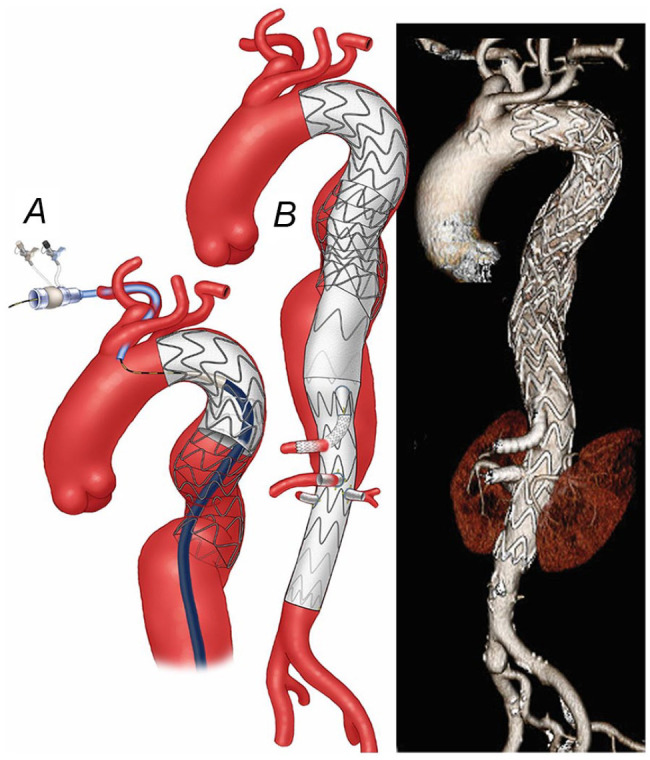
(A) Once the wire was confirmed to be in the central lumen of the stent, the fenestrated-branched stent-graft with a directional branch for the celiac axis and 3 fenestrations for the superior mesenteric artery and renal arteries was successfully deployed. (B) Postoperative 3-dimensional computed tomography angiography reconstruction shows the endovascular repair of an extent II thoracoabdominal aortic aneurysm with no kink, stent compressions, and endoleak.
